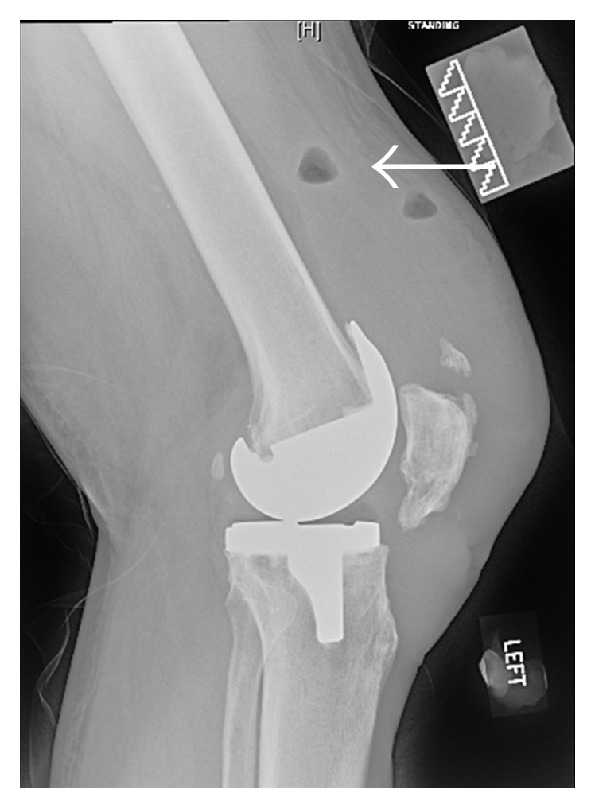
Lateral left knee X-ray showing a patella baja, fluid collection near the quadriceps tendon, and the presence of gas in the thigh, with query infection.
